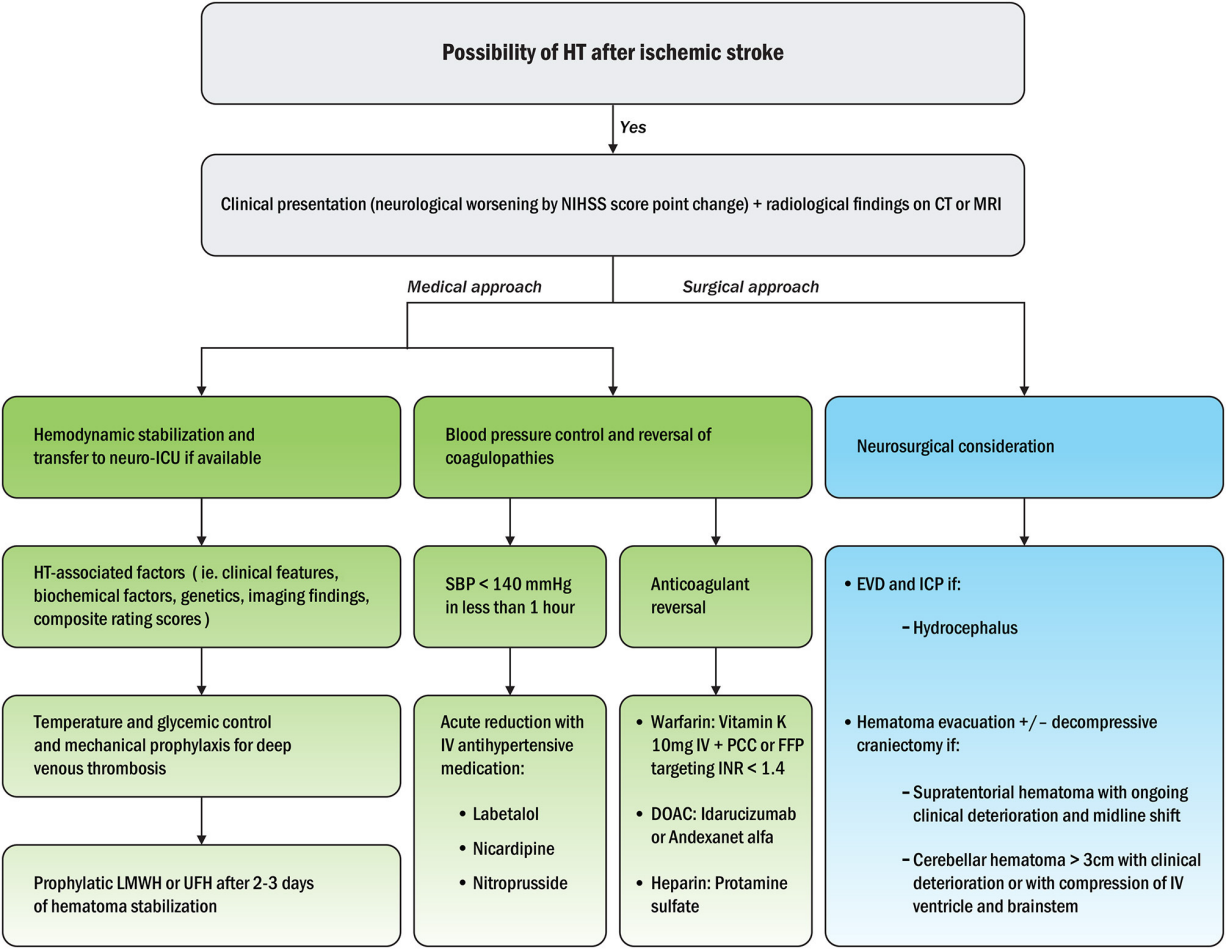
Treatment algorithm for appropriate medical and surgical approaches to HT after ischemic stroke (115). NIHSS, National Institutes of Health Stroke Scale; CT, computed tomography; MRI, magnetic resonance imaging; ICU, intensive care unit; LMWH, low molecular weight heparin; UFH, unfractionated heparin; SBP, systolic blood pressure; IV, intravenous; PCC, prothrombin complex concentrates; FFP, fresh frozen plasma; INR, international normalized ratio; DOAC, direct oral anticoagulants; EVD, external ventricular drainage; ICP, intracranial pressure.
